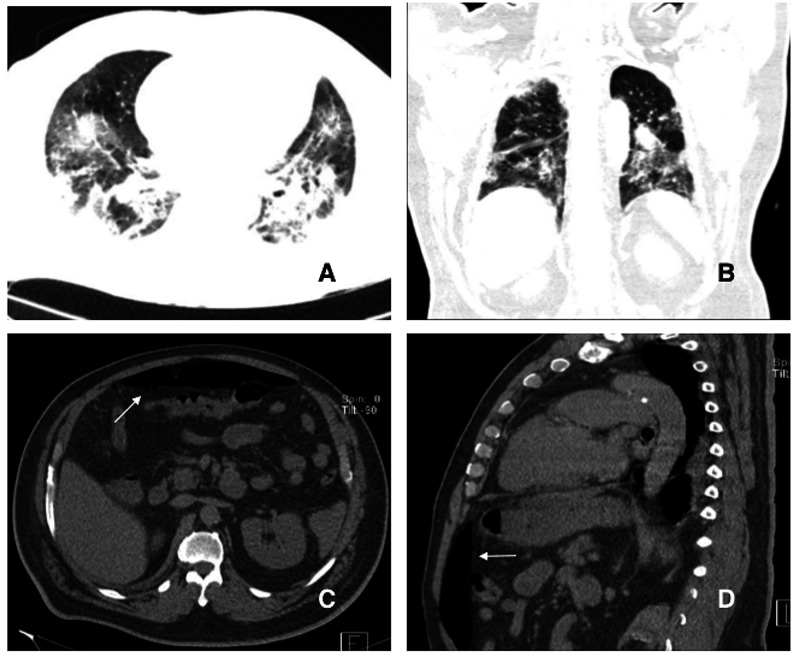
CT images of the chest and abdomen. Panels A and B: Diffuse ground-glass opacities in both lungs; Panels C and D: Abdomen images showing pneumoperitoneum (arrows).
